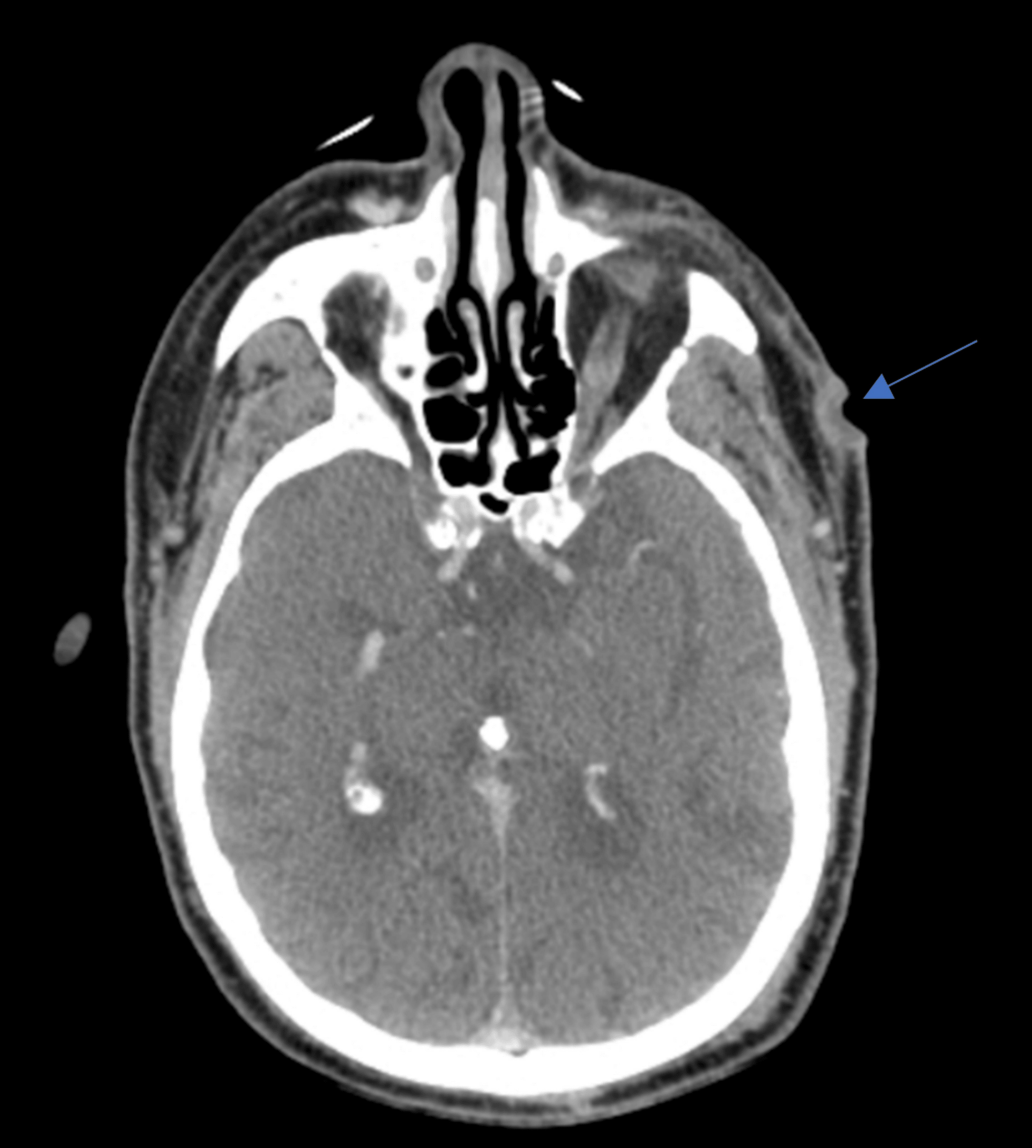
Staging CT scan. CT scan from March 2021 revealing a left malar lesion with cutaneous and subcutaneous thickening and ulceration. No distant metastasis was seen.
